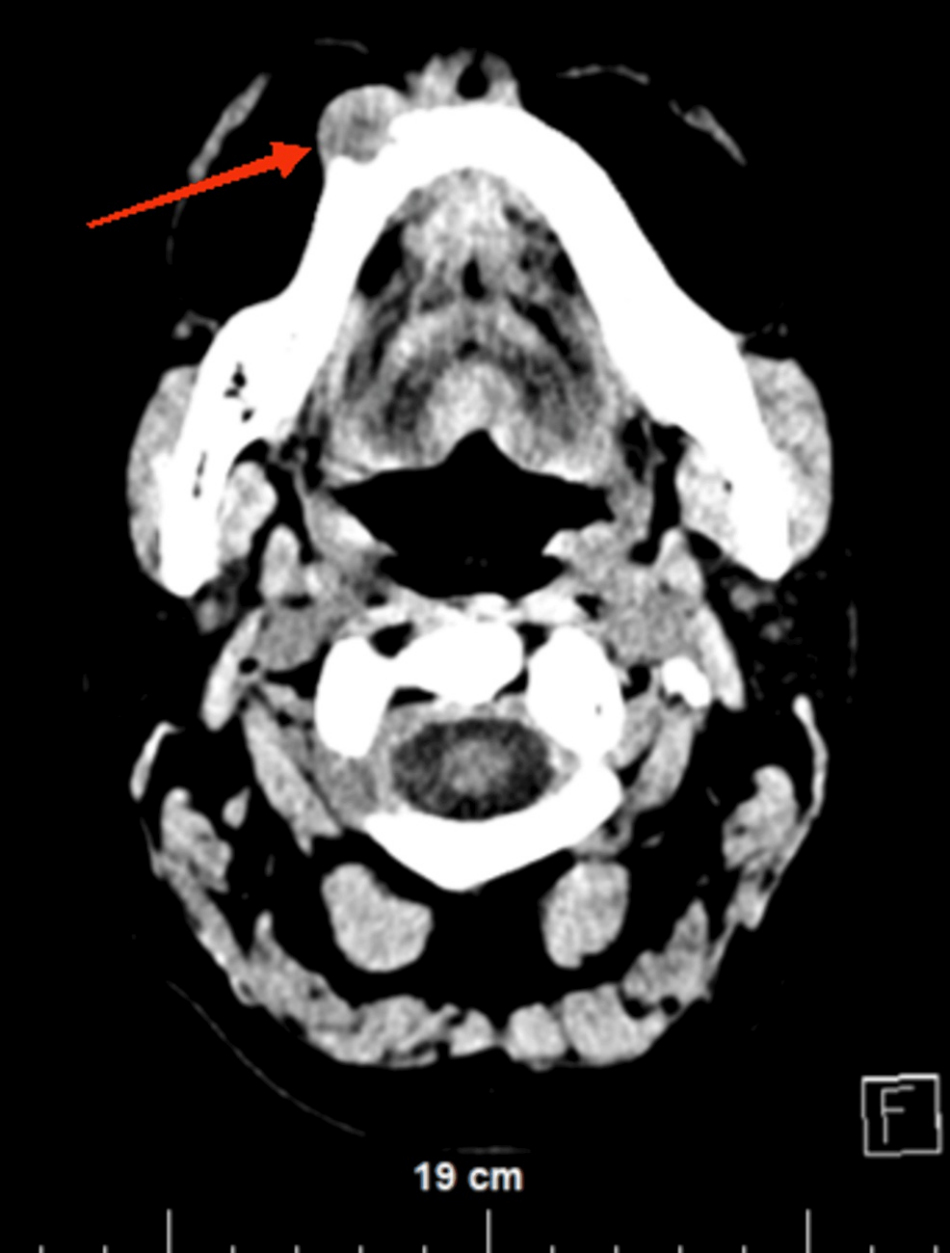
Coronal section from a native CT scan of the head showing the tumor invasion in the alveolar process of the right mandible (red arrow)
